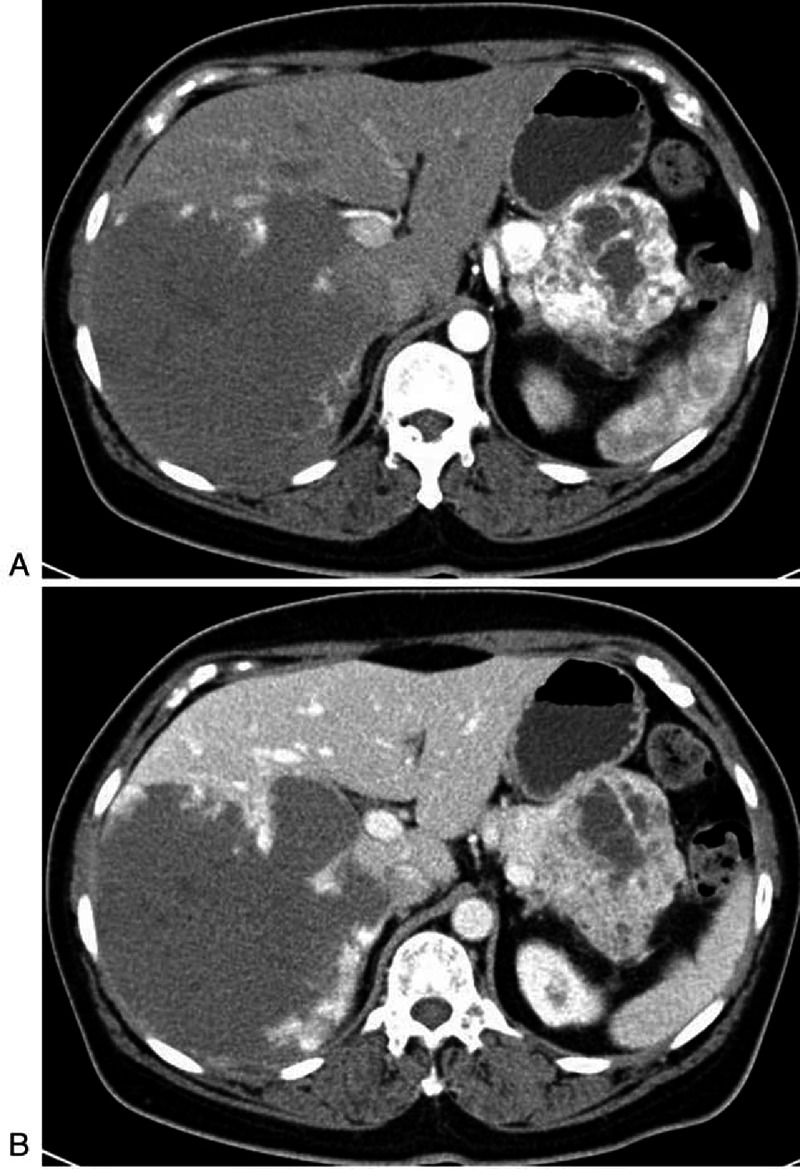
A 62-year-old woman with RCC (patient 9). A, Arterial phase image from contrast-enhanced CT depict two hypervascular masses (close to together) in the pancreatic tail. The small one has homogeneous enhancement, while larger lesion has heterogeneous enhancement with many hypodense areas consistent with necrosis. B, The two masses wash out on the venous phase, similar to RCC. A giant hemangioma in the liver is also seen.
